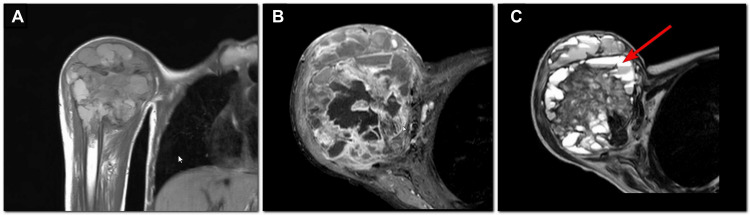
MRI right shoulder joint, (A) T1 coronal, (B) T1 postcontrast axial, and (C) T2 axial images. Follow-up scan after two cycles of chemotherapy. The mass shows posttreatment necrosis, seen as hyperintense areas with fluid-fluid levels on T2 images (arrow in C)
